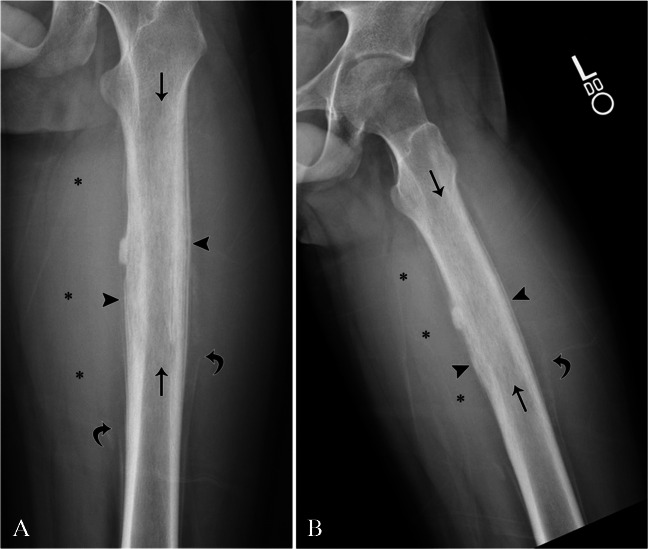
17-year-old male with Ewing sarcoma of the left femur. Anteroposterior (AP) (A) and lateral (B) radiographs demonstrate a permeative lytic lesion (arrows) with a combination of "onion peel" periosteal reaction (arrowheads) and Codman's triangle (curved arrows). A large extraosseous component can be extrapolated from marked adjacent soft tissue thickening (*)
